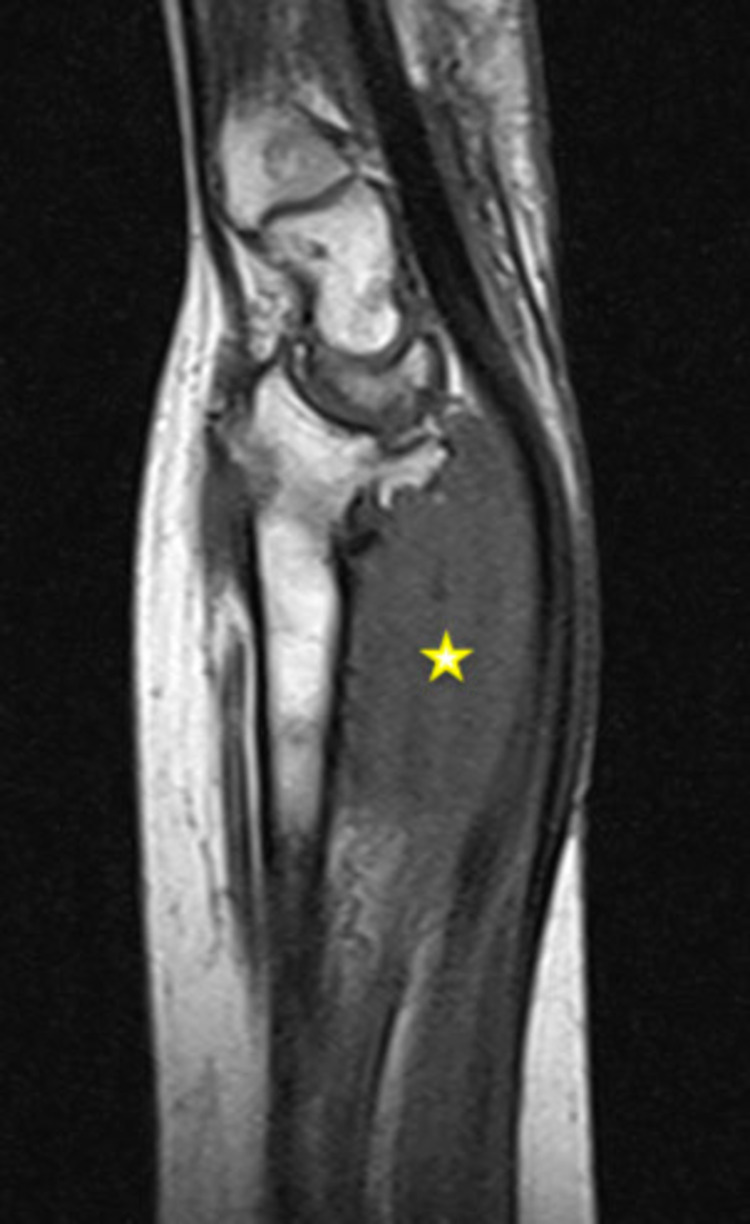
Coronal view of the left wrist (MRI; T1W image). The lesion (yellow asterisk) shows a homogenous low signal, slightly lower to the adjacent muscle. MRI: magnetic resonance imaging; T1W: T1-weighted
